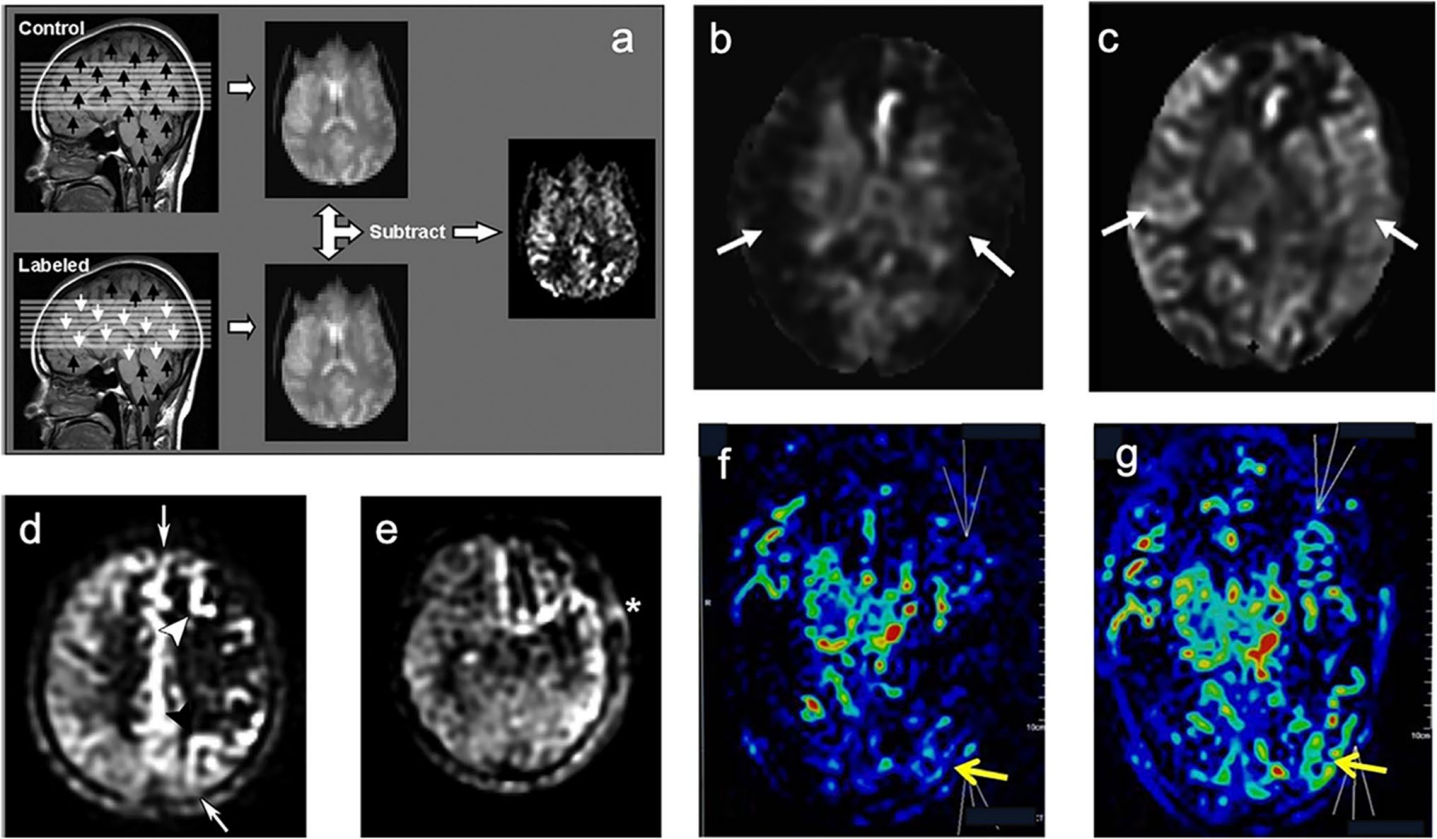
Arterial spin-labeled MRI in moyamoya disease. a Blood proton from the ICA was labeled once (labeled) and twice (controlled) by 180° radiofrequency pulse, respectively. After T1 delay, the protons arrived at the signal acquisition area, and the labeled image and control image were obtained respectively. After subtraction of these two images, a difference image with intensity proportional to cerebral blood flow (CBF) was obtained, i.e., cerebral perfusion image. b, c ASL sequences of a moyamoya patient with good recovery after bilateral bypass surgery. The preoperative ASL (b) signal (*arrows*) war dark, indicating markedly reduced perfusion to bilateral MCA territories. The postoperative image (c) shows increased ASL signal in the bilateral MCA territories (*arrows*). d, e ASL sequences of a moyamoya patient with poor recovery after left bypass surgery. Preoperative ASL images (d) show decreased perfusion (arrows) and arterial transit artifact (ATA) signals in left distal ICA territories (arrowheads) indicating late-arriving flow via collateral pathways. After left MCA–STA bypass surgery, postoperative ASL images (e) still show impaired perfusion and ATA signals in left MCA territory and prominent ATA signal in MCA–STA anastomosis site ( ∗) indicating flow stagnation in anastomosis site. f, g Color-coded ASL perfusion images of a moyamoya patient who had moderate to severe headache with seizures at the second postoperative day, and was clinically suspected as hyperperfusion syndrome. There was a significantly raised perfusion (more than 100% increase in CBF values) in the left occipital regions (yellow arrows) between preoperative (f) and postoperative (g) [39, 49, 56, 72]
